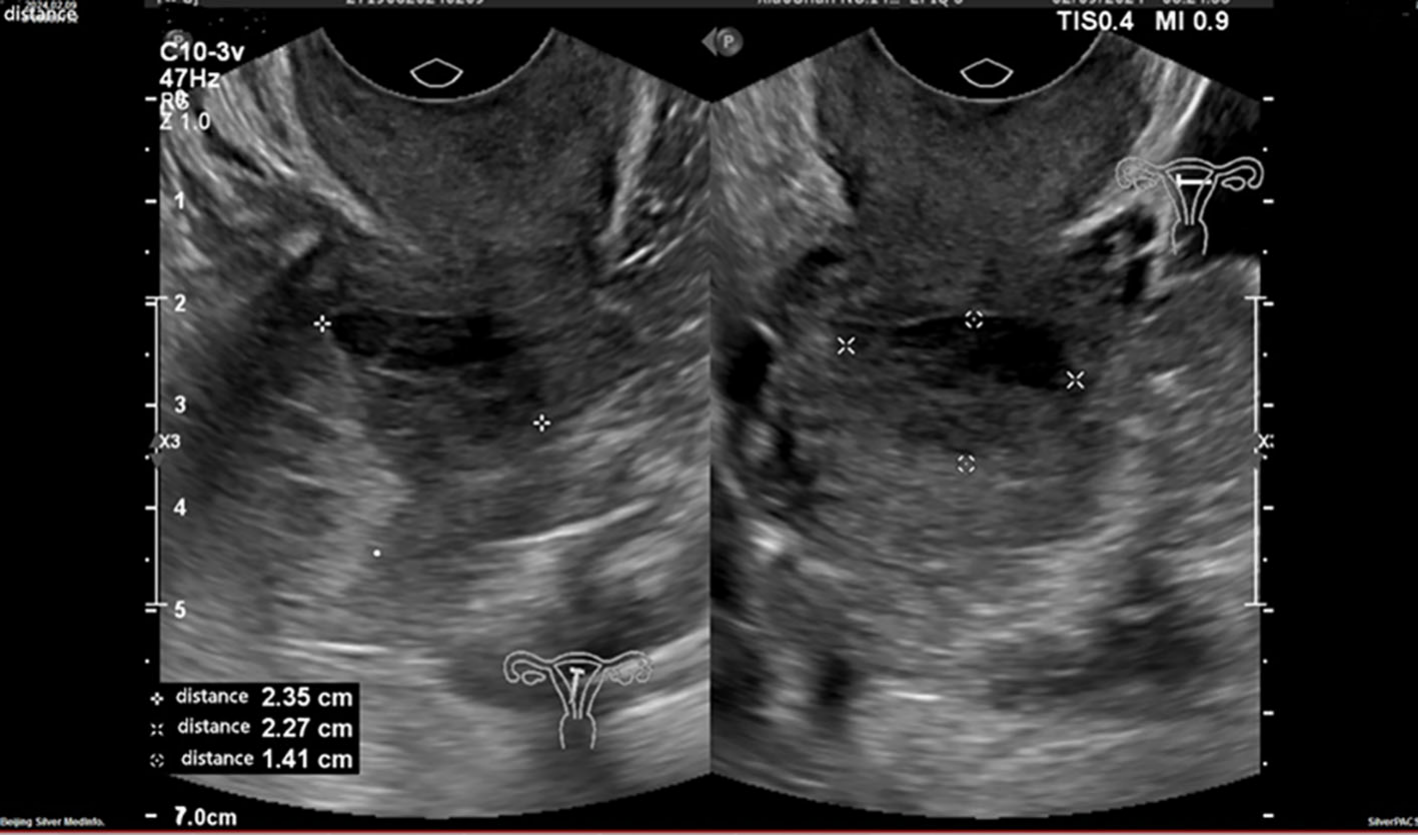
An ultrasound examination reveals a hypoechoic mass in the left posterior wall of the uterus.
